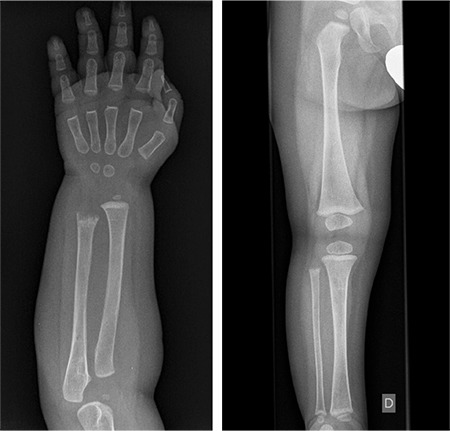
Radiographs at the age of 14 months
